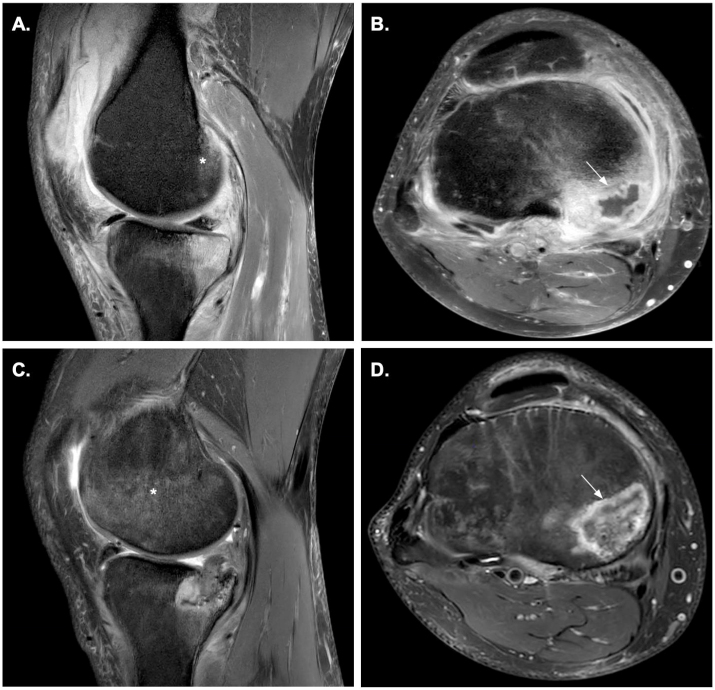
Magnetic resonance imaging (MRI) of the right knee with and without contrast acquired on day +49 before surgery (A. and B.), and day +195 after initial debridement in the joint space and the medial tibial plateau (B. and C.). Fat suppressed proton density (FS-PD) MRI sequences demonstrating osteomyelitis within the lateral femoral condyle (Labeled with “*”; A. and C.). T-2 weighted MRI sequences demonstrating medial tibial plateau osteomyelitis with abscess 2.3 × 1.3 × 0.3cm, and post-debridement changes (Labeled with arrows; B. and D., respectively).
